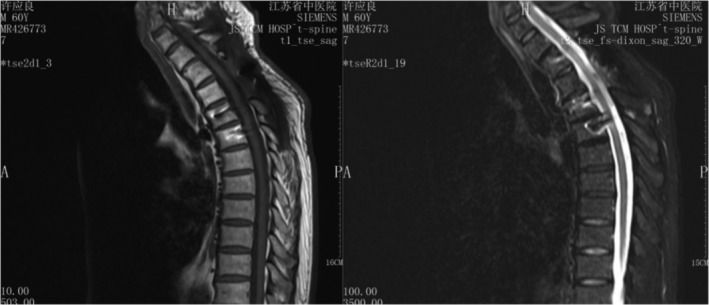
MRI and CT scan after SBRT precision radiotherapy (3 months).
